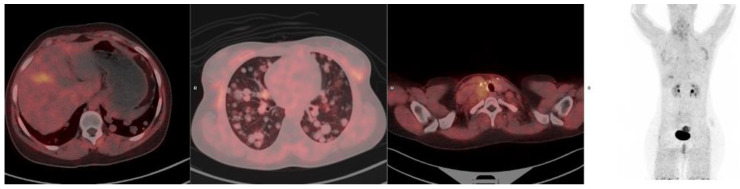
^68^Ga-FAPI-46 PET/CT images of a 19-year-old female with metastatic papillary thyroid cancer (follicular variant). In addition to the cervical and lymph node metastases, false positive uptake is seen in the gallbladder on ^68^Ga-FAPI-46.
